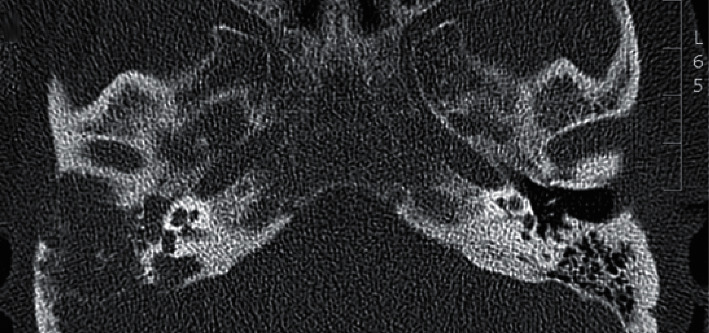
Axial view of the initial temporal CT showed complete opacification of the external auditory canal, middle ear, and mastoid on the right side indicating a soft tissue mass.
